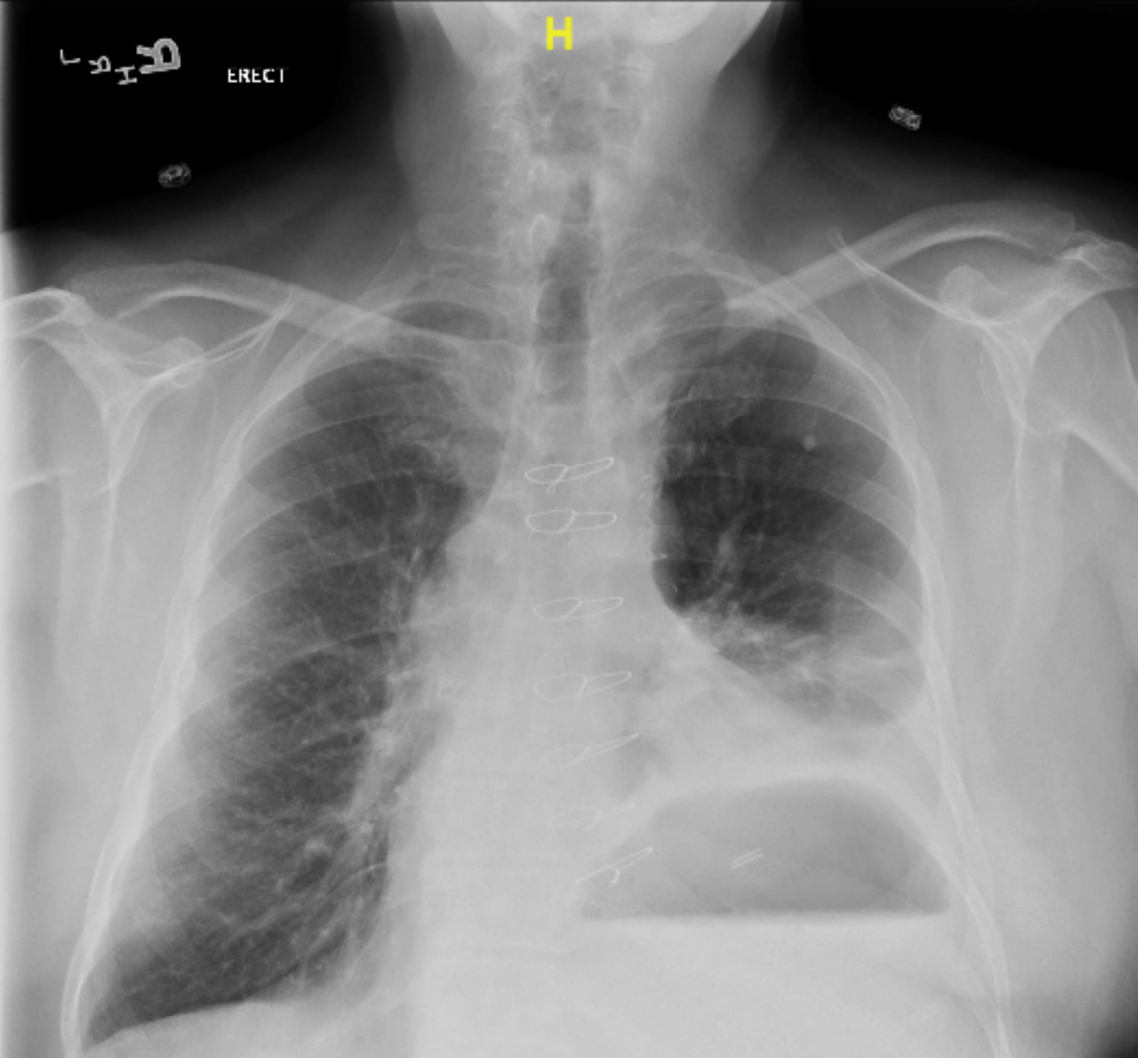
A CXR revealed a left lower lobe infiltrate. CXR, chest X-ray
